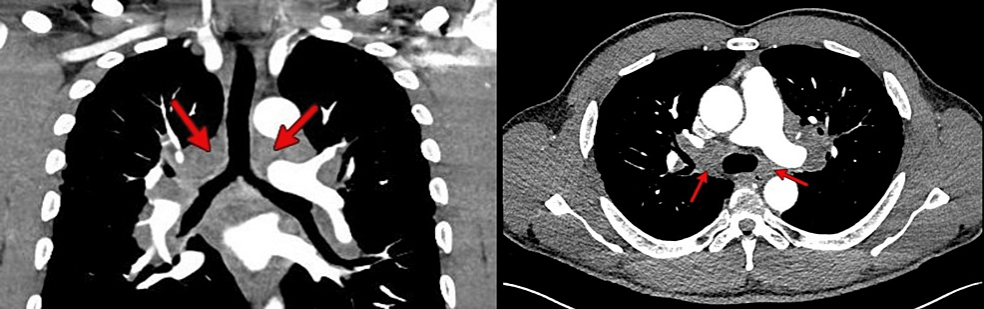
Chest CTA showing bilateral hilar and mediastinal lymphadenopathy with bilateral pulmonary perilymphatic nodules in cross-sectional (left) and transverse (right) views. CTA: computed tomography angiography
